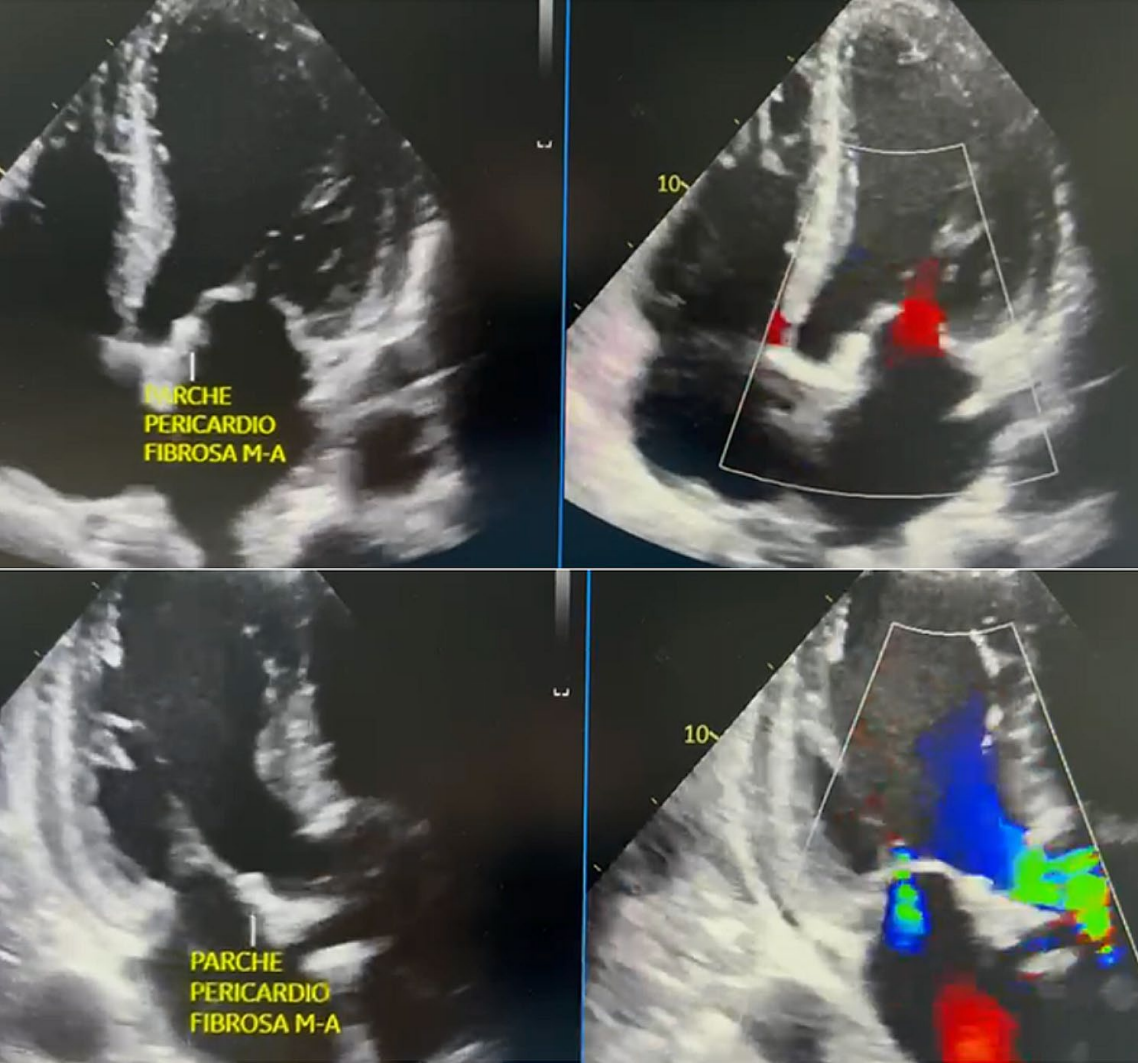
Postoperative transesophageal echocardiogram (A) Four-chamber apical view. Evidence of pericardial patch in the mitro-aortic fibrous portion occluding the fistulous tract, with no flow. (B) Two-chamber apical view. Evidence of pericardial patch in the mitro-aortic fibrous portion occluding the fistulous tract, with no flow.
